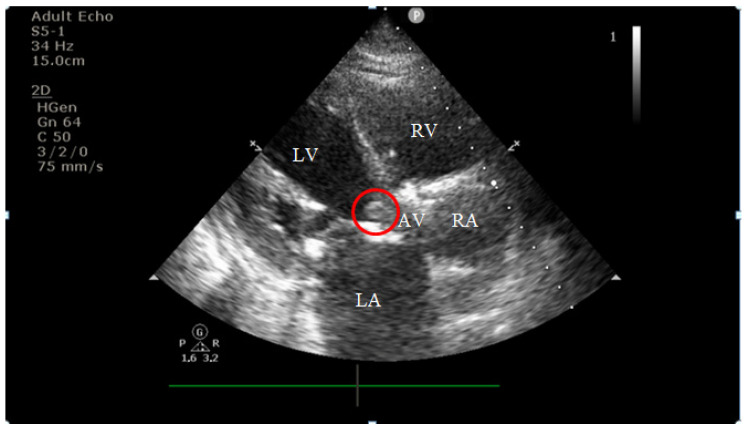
Echocardiography on admission showing a hyperechogenic, mobile, irregular mass attached to the aortic prosthetic valve; LV—left ventricle; RV—right ventricle; LA—left atrium; RA—right atrium; AV—aortic valve; red circle—hyperechogenic, mobile, irregular mass attached to the aortic prosthetic valve, measuring 8 × 16 mm.
